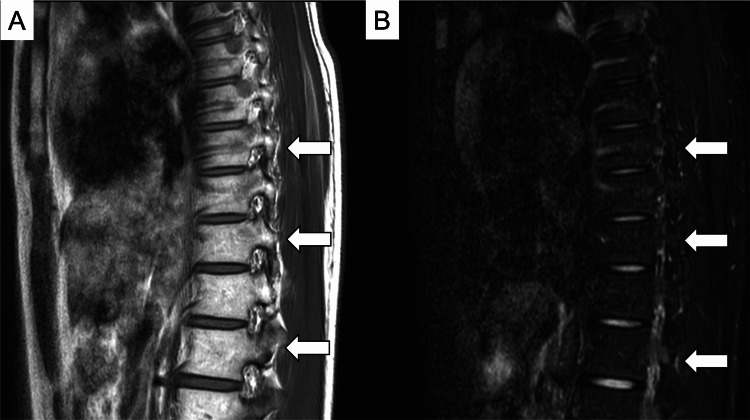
Magnetic Resonance Imaging (MRI) of the Thoracic Spine T1-weighted (A) and short-tau inversion recovery (STIR) images (B) demonstrating high T1 and low STIR signal intensity, indicative of fatty marrow infiltration compatible with aplastic marrow phenotype.
